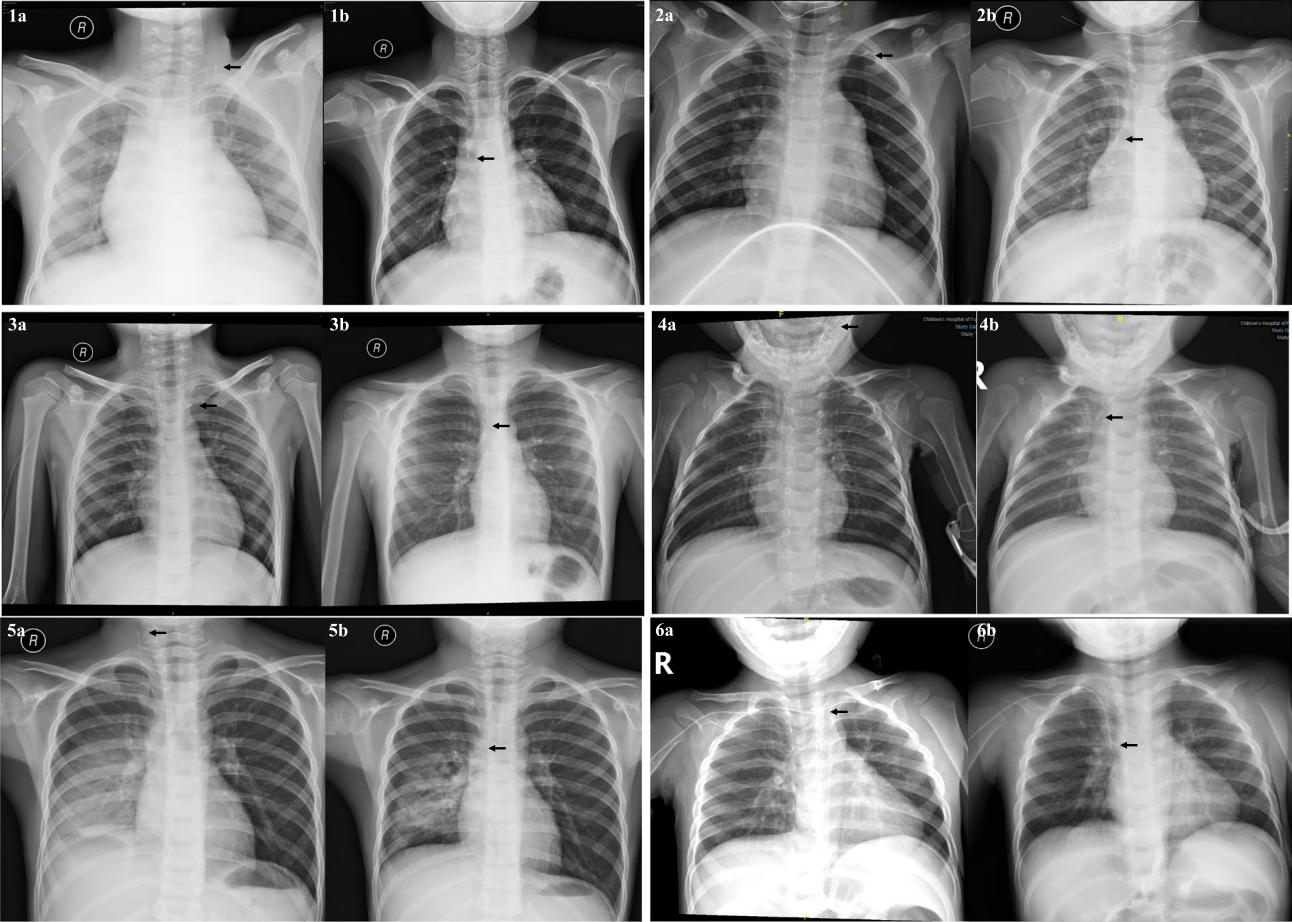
X-ray images of PICC before(a) and after arm movements(b). 1a/1b, 13-year boy, right basilic vein insertion, 4.0 Fr catheter, the tip was in the right jugular vein before arm movements. 2a/2b, 6-year girl, right basilic vein insertion, 4.0 Fr catheter, the tip was in the left subclavian vein before arm movements. 3a/3b, 5-year boy, right cephalic vein insertion, 4.0 Fr catheter, the tip was in the left subclavian vein before arm movements. 4a/4b, 5-month boy, left axillary vein insertion, 1.9Fr catheter, the tip was in the left jugular vein before arm movements. 5a/5b, 7-year boy, right cephalic vein insertion, 3.0 Fr catheter, the tip was in the right jugular vein before arm movements. 6a/6b, 20-month boy, right basilic vein insertion, 3.0 Fr catheter, the tip was in the left brachiocephalic vein before arm movements. Tips of all PICCs were in the superior vena cava after arm movements
